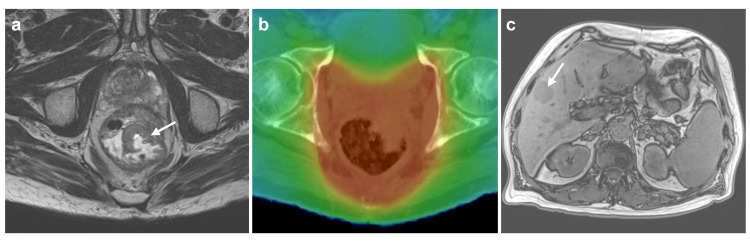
Case example Case example of a 69-year-old patient with UICC stage IIIC LARC, who underwent SC-TNT and R0 resection. (a) MRI showing the initial extension of the LARC (arrow). (b) Dose distribution of volumetric modulated arc therapy (axial image). (c) MRI demonstrating a singular liver metastasis (arrow), diagnosed approximately three months after surgery, after the patient complained about upper abdominal pain. UICC: Union for International Cancer Control; LARC: Locally advanced rectal cancer; SC: short-course; TNT: total neoadjuvant therapy
